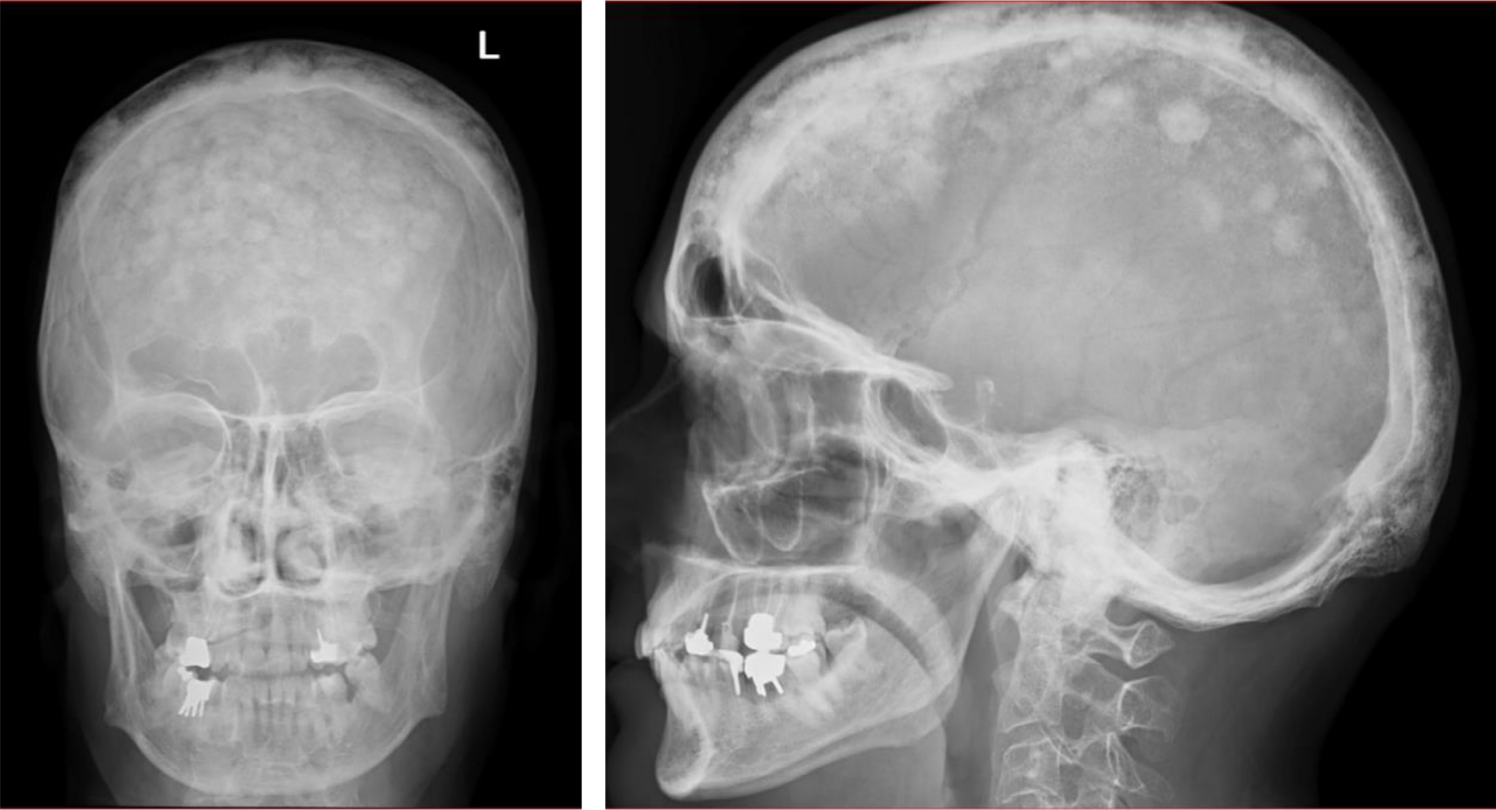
Plain radiography of skull 20 month after treatment showing marked improvement of lytic and fibrotic lesions.
